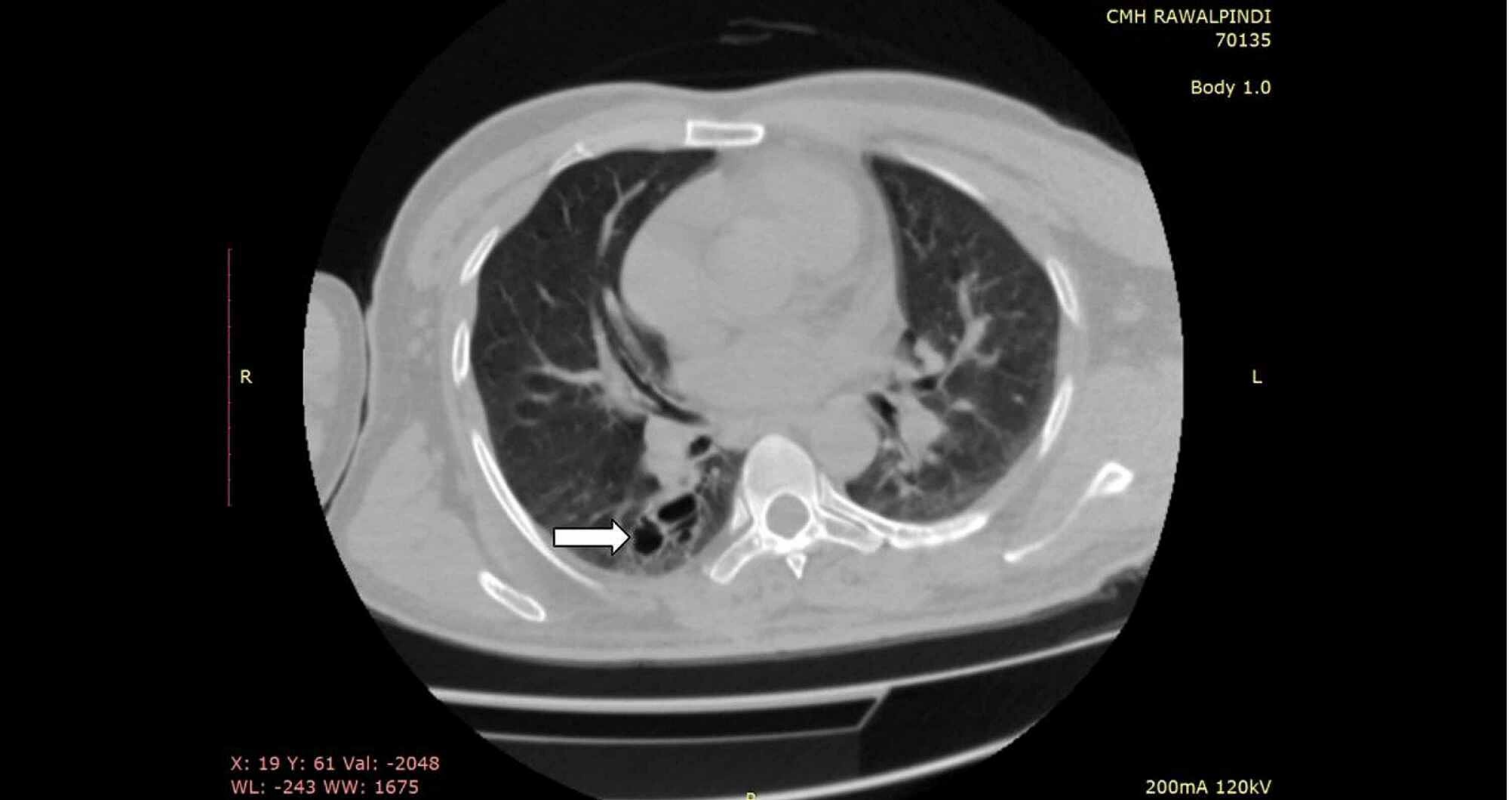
Axial thoracic HRCT shows a moderately bronchiectatic right lung, considered sequelae of previous tuberculosis cystic bronchiectasis in the superior segment of the right lower lobe (arrow).
